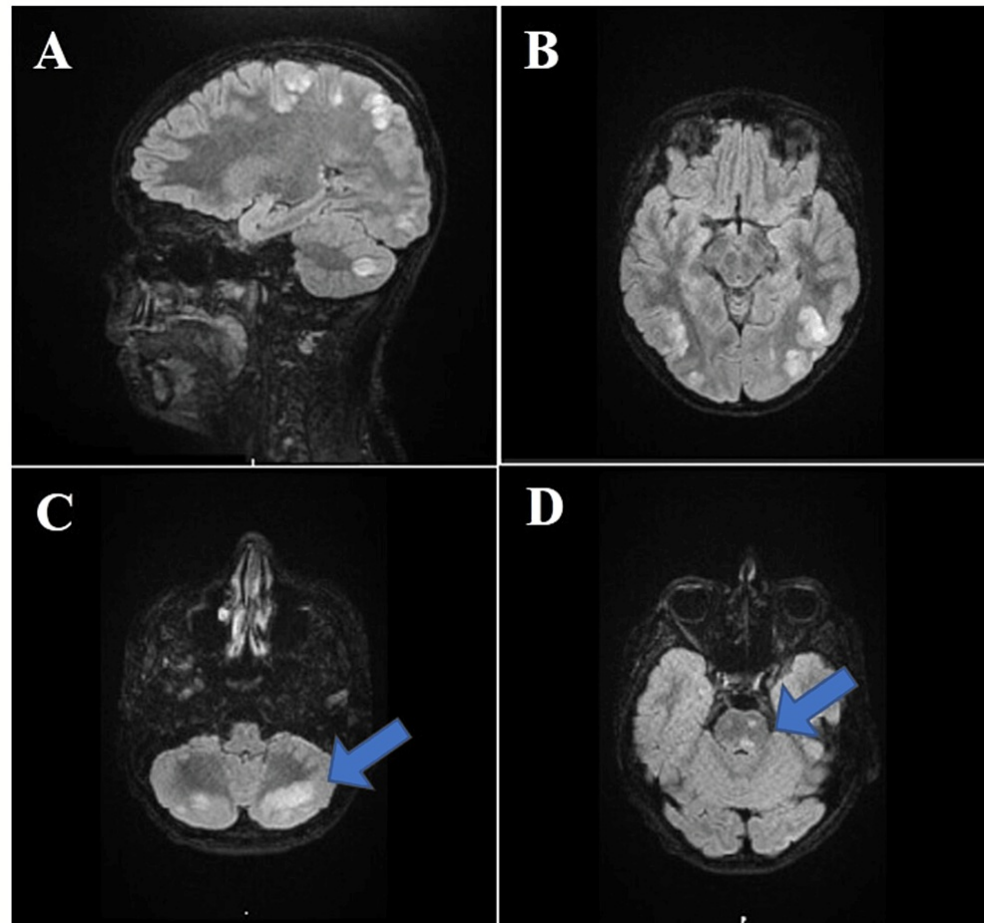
MRI findings consistent with PRES (patient 1). Multiple high-intensity lesions in cortical and subcortical white matter on the parieto-occipital and posterior frontal regions (images A and B), posterior area of cerebellar hemispheres (image C, arrow), and pontomesencephalic transition (image D, arrow).
